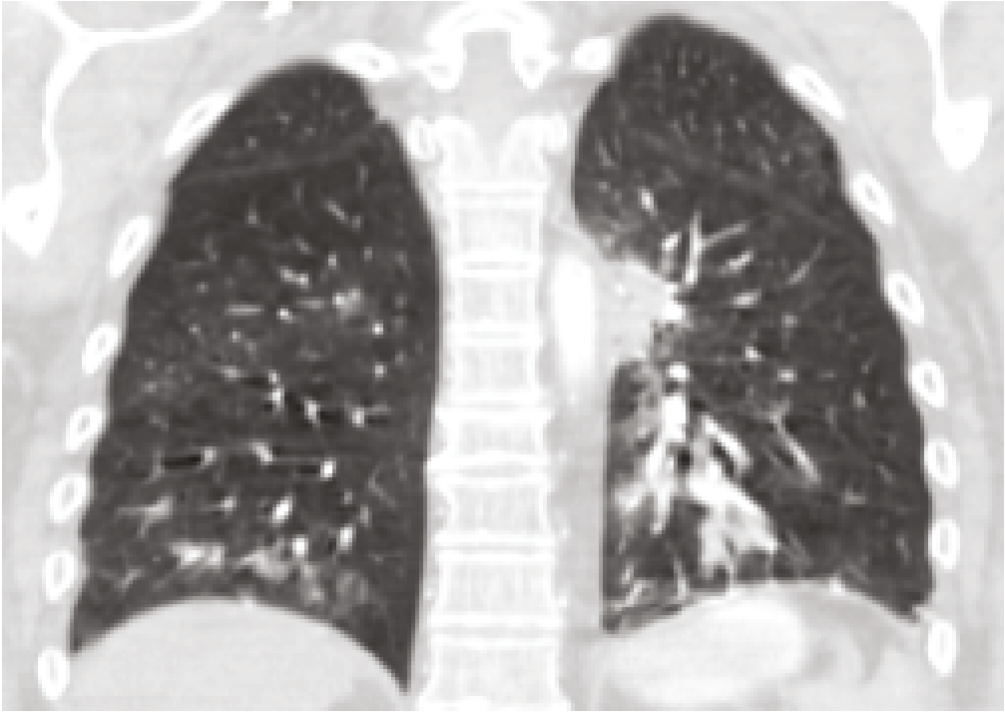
CT scan of the chest showing bilateral ground glass opacities with a left-sided hilar and lower lobe infiltrate.
